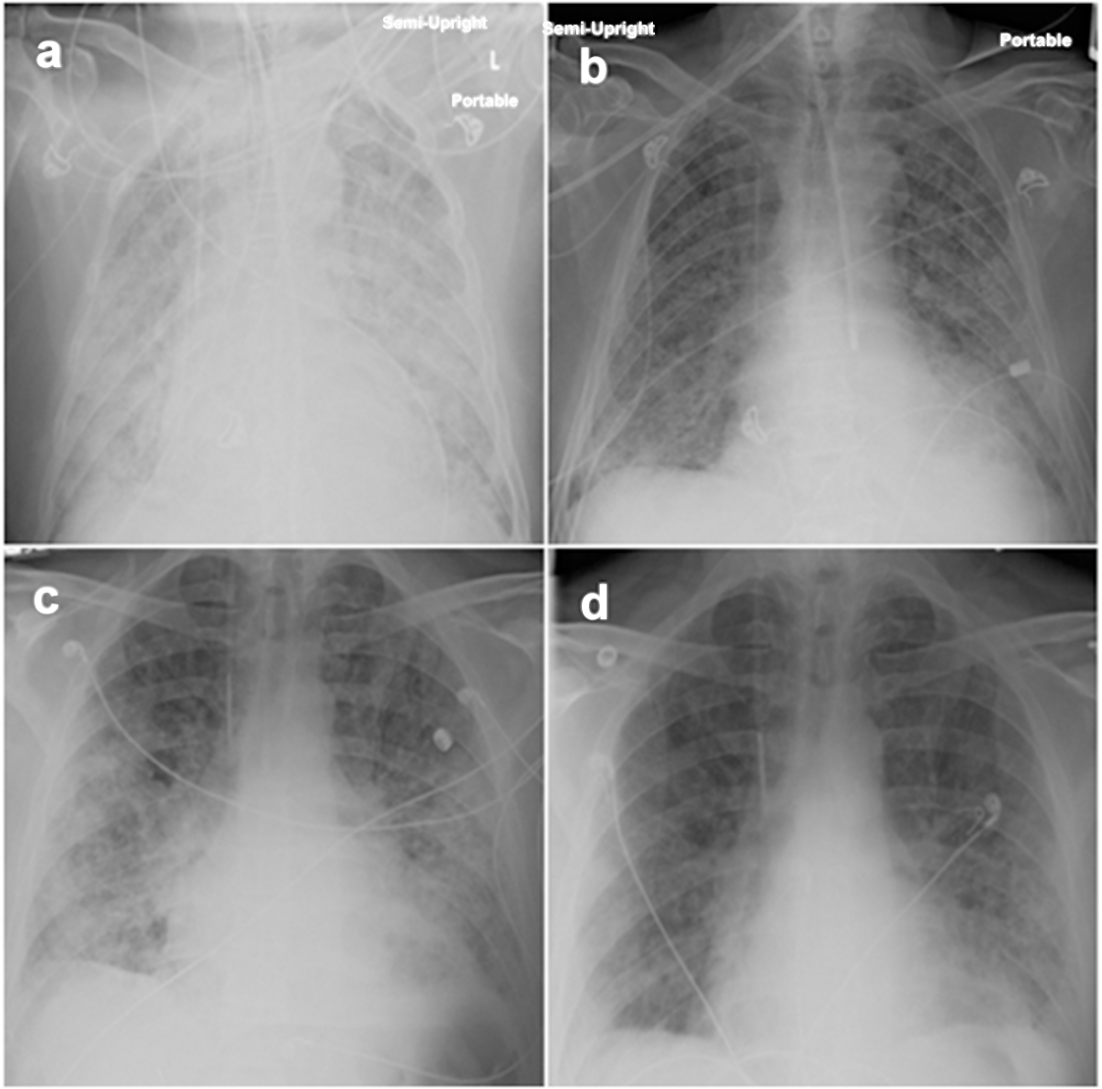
Radiographic Changes with Experimental Treatment. In addition to better lung function and gas exchange, experimental treatments frequently improved the chest radiographs (CXR) of these subjects. a) Pretreatment CXR of Subject #5 during relapse shows diffuse infiltrates; b.) Radiographic improvement (and extubation) of this subject to 2^nd^ TPE series. c.) CXR of Subject #7 immediately prior to treatment and d.) after first three TPE. These (and other) radiographic and clinical improvements in the subject population were not attributable to changes in intravascular volume status or infection.
